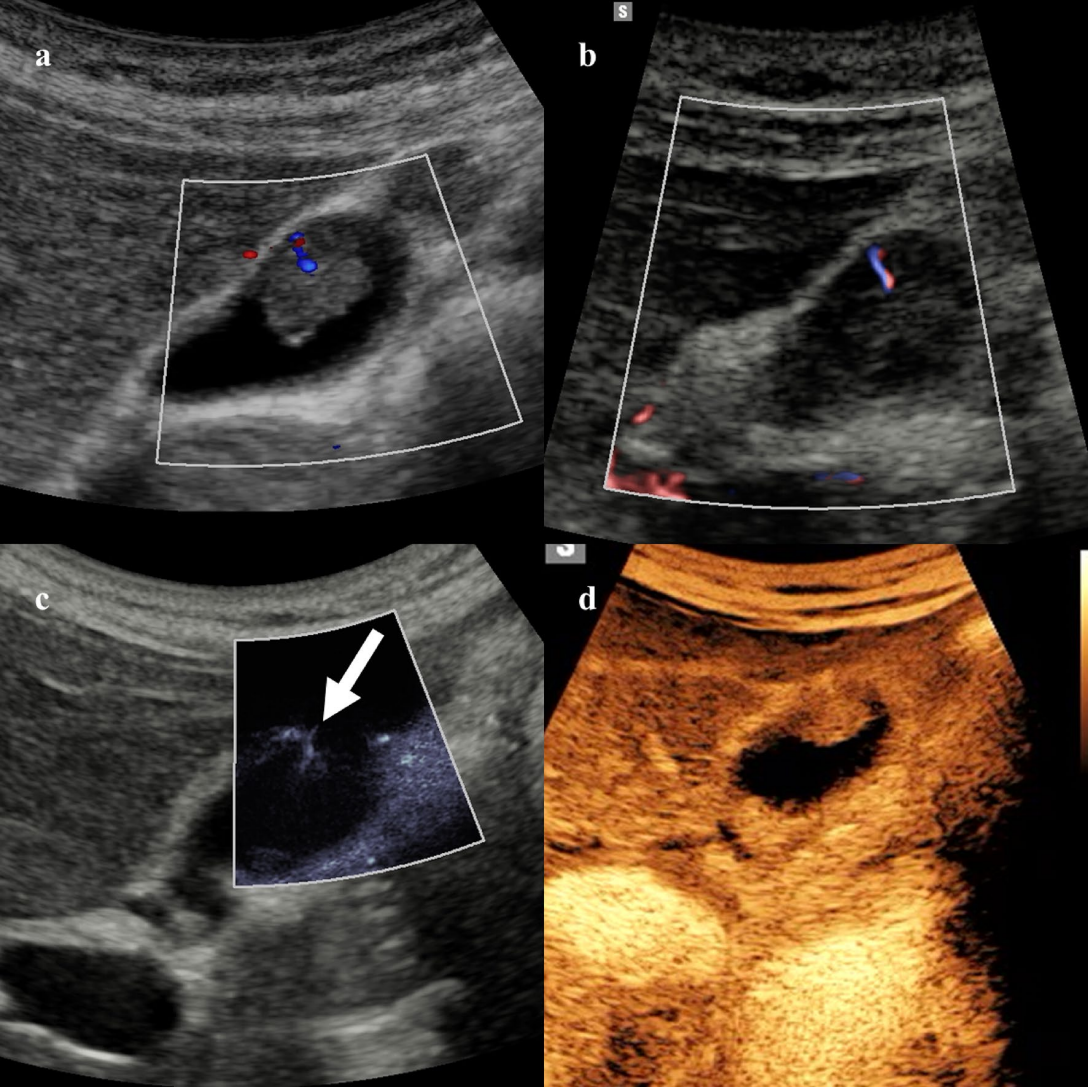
49-year-old woman with incidentally detected 1.2 cm gallbladder polyp. Color (a), directional power Doppler (b), and microvascular flow imaging (c) demonstrate intralesional vascular signal, with branching appearance on MVUS (arrow). The lesion shows homogeneous enhancement on contrast-enhanced ultrasound at 32 s after contrast injection (d). This lesion was confirmed as an adenomatous polyp after cholecystectomy
